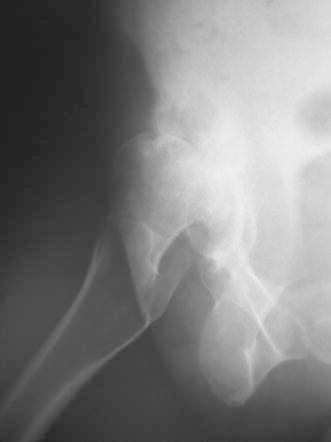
Preoperative radiographic aspect in axial view
